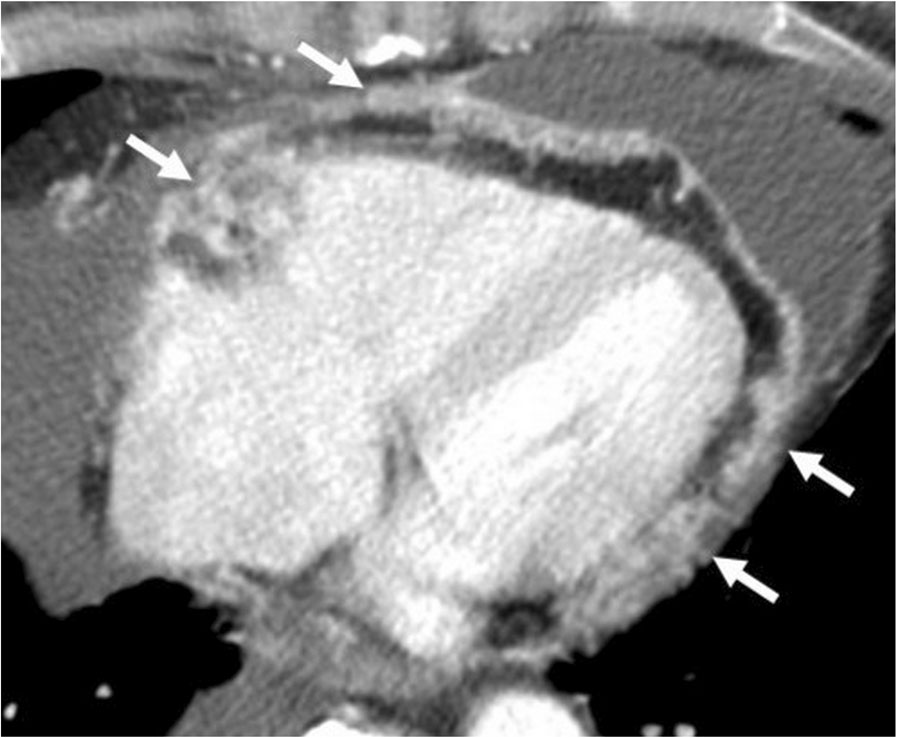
A 48-year-old woman with pathologically proven renal cell carcinoma. Axial contrast-enhanced CT image demonstrates the diffuse irregular thickening and contrast enhancement of the pericardium (arrows) compatible with pericardial metastasis
